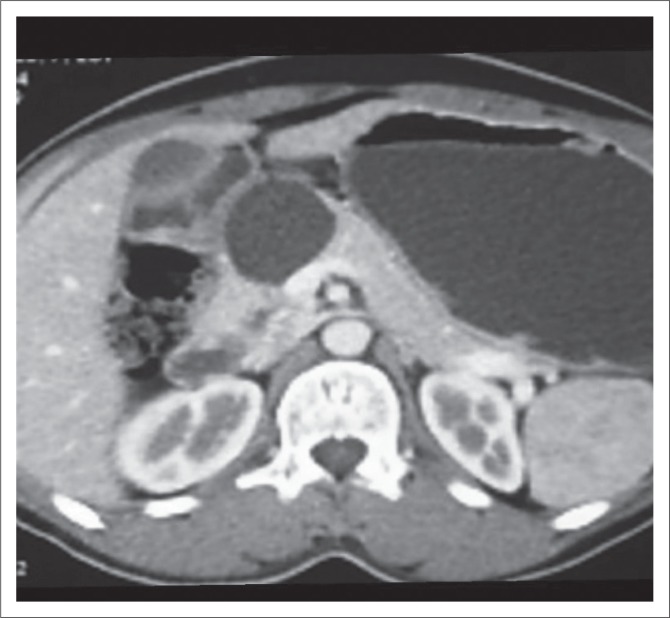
Axial contrast-enhanced computed tomography image in a 25-year-old male patient showing a simple pancreatic cyst. No septation or wall enhancement seen. Main pancreatic duct is not dilated.
